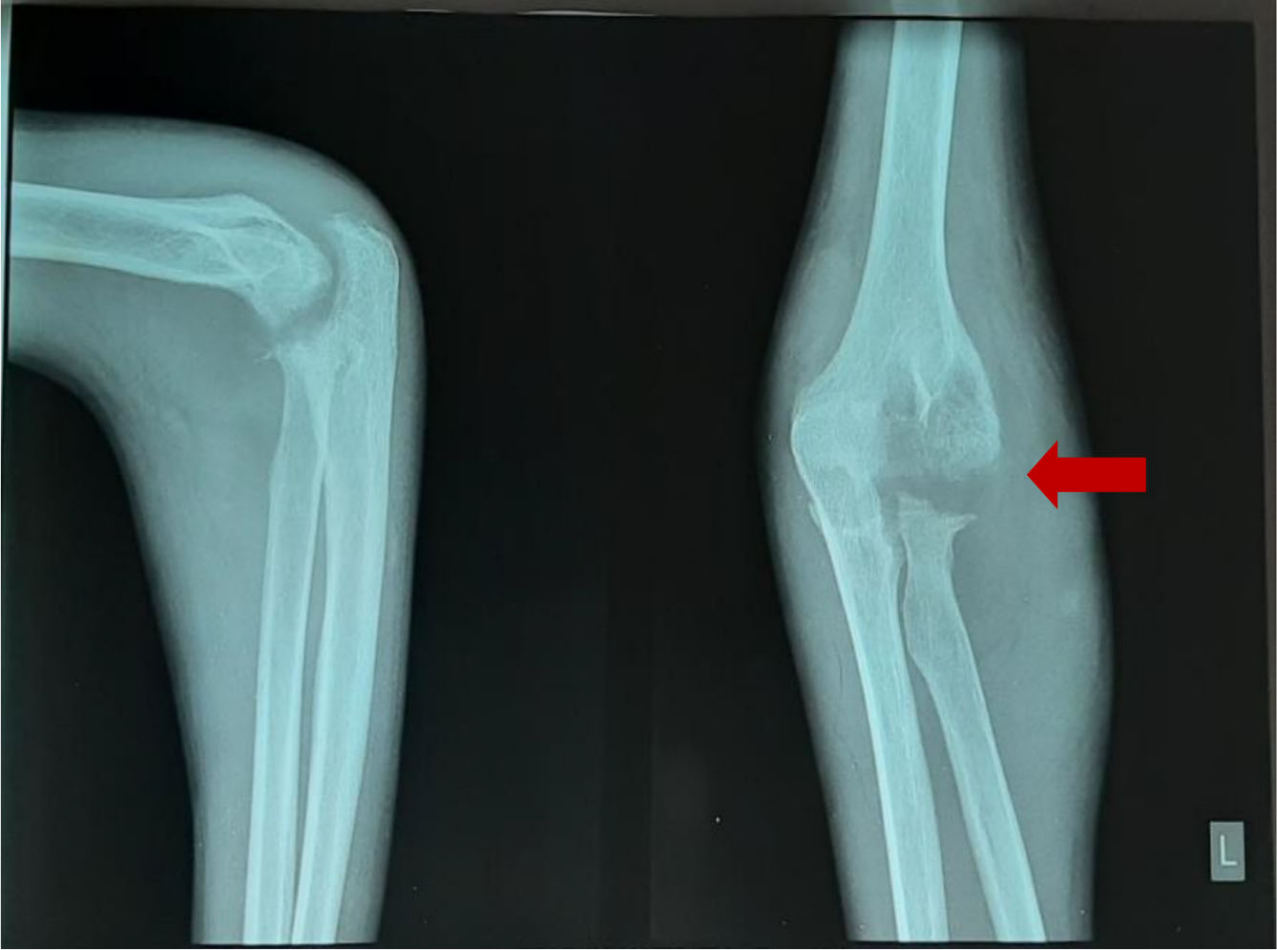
Minimal improvement, AP and lateral projection of the left elbow joint radiographs showed erosion of the distal cortex of the humerus and radial bone, destruction of the distal cortex, and swelling of the soft tissue of the left elbow area (March 2021).
